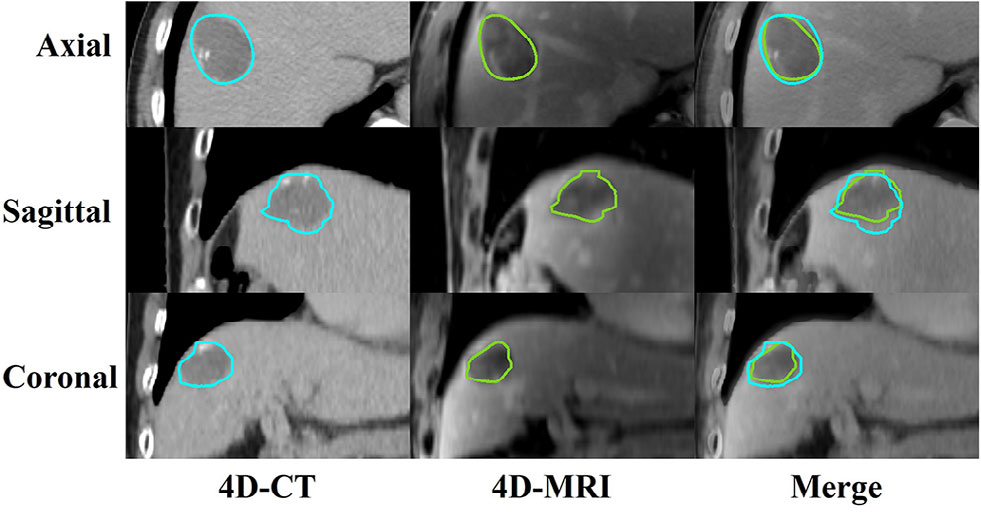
Images of GTV in liver cancer patients. Multiplanar (axial, sagittal, and coronal planes) four‐dimensional computed tomography (4D‐CT), four‐dimensional magnetic resonance imaging (4D‐MRI), and fused imaging datasets acquired at the end‐expiration phase from a selected patient case.
